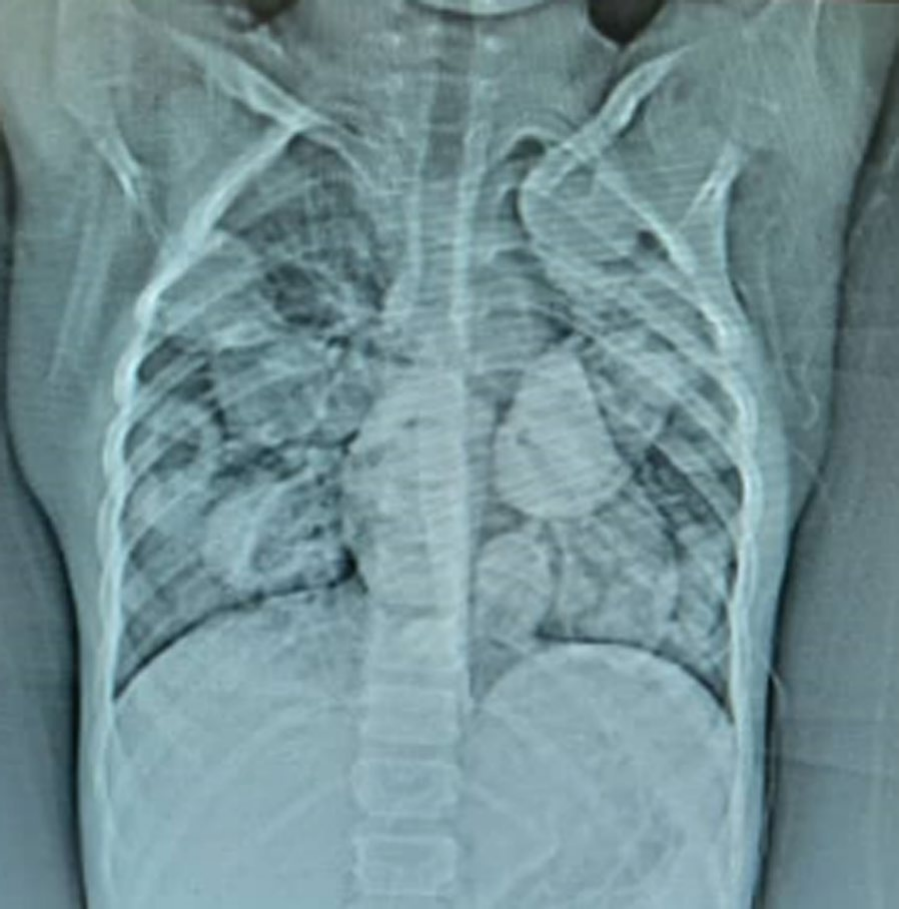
Plain chest X-ray at AP view shows multiple bilateral areas of consolidation
